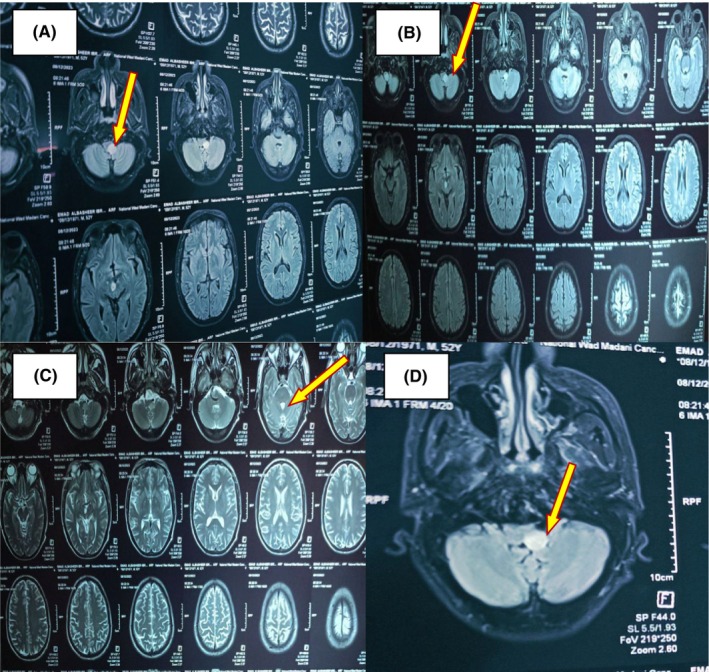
(A–D) Brain MRI showed 1.4 × 1.3 cm low T1 and high T2 right lateral cerebello‐pontomedullary ischemic lesion exert homogenous post‐contrast enhancement.
